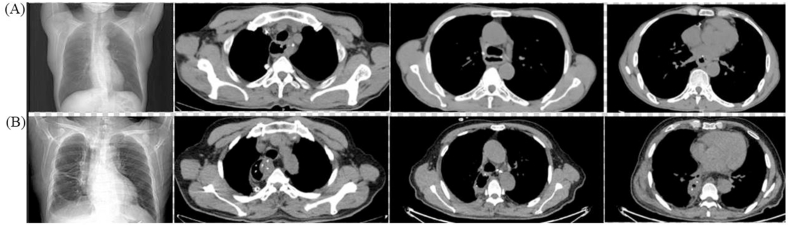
Posterior-mediastinal route of the gastric tube. (A) Retrocardiac orthotopic route (original esophageal bed); (B) Intrathoracic heterotopic route. From left to right: chest radiograph, level below the thoracic inlet, azygos arch level, inferior pulmonary vein level.
